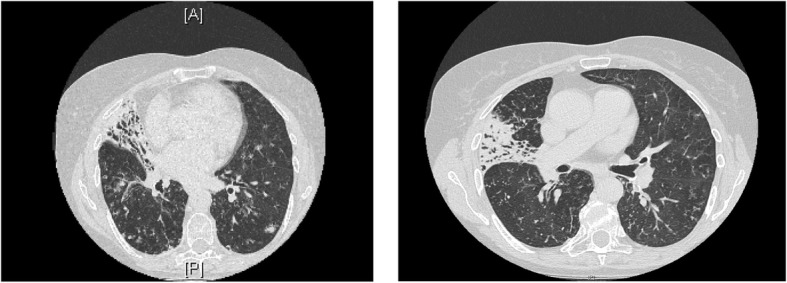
CT Thorax 2016: High resolution CT Chest from 2016 demonstrating extensive airspace opacification, ill-defined nodularity and tree in bud change
